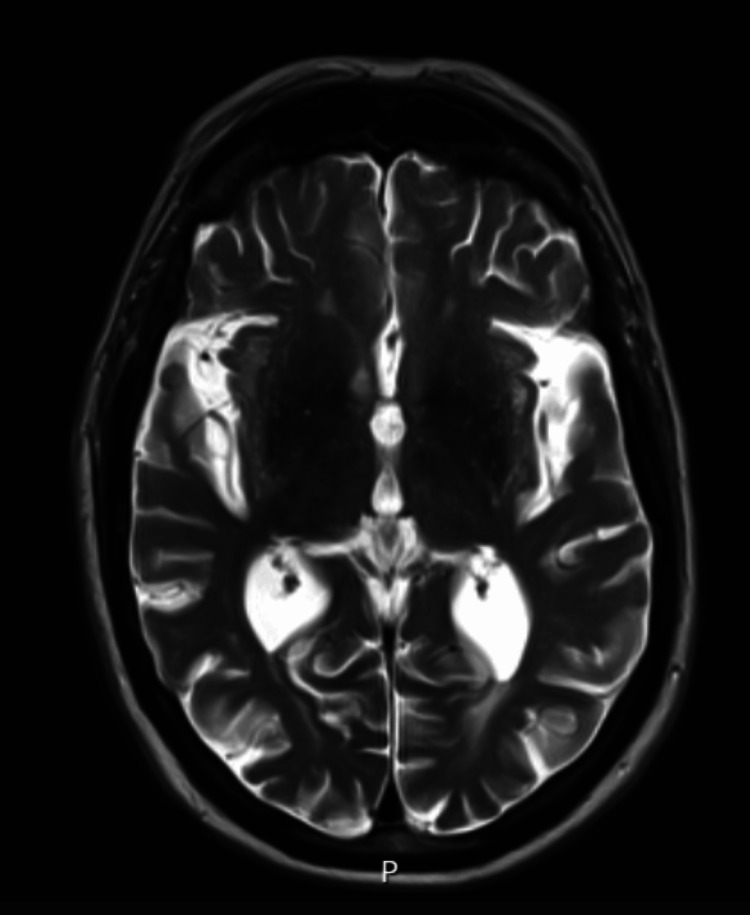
Axial T2-weighted MRI corresponding to reported cortical, ventricular, and hippocampal changes Axial T2-weighted MRI from the patient’s scan, demonstrating widened cortical sulci and ventricular prominence, with hippocampal region volume loss as reported in the radiological assessment.
